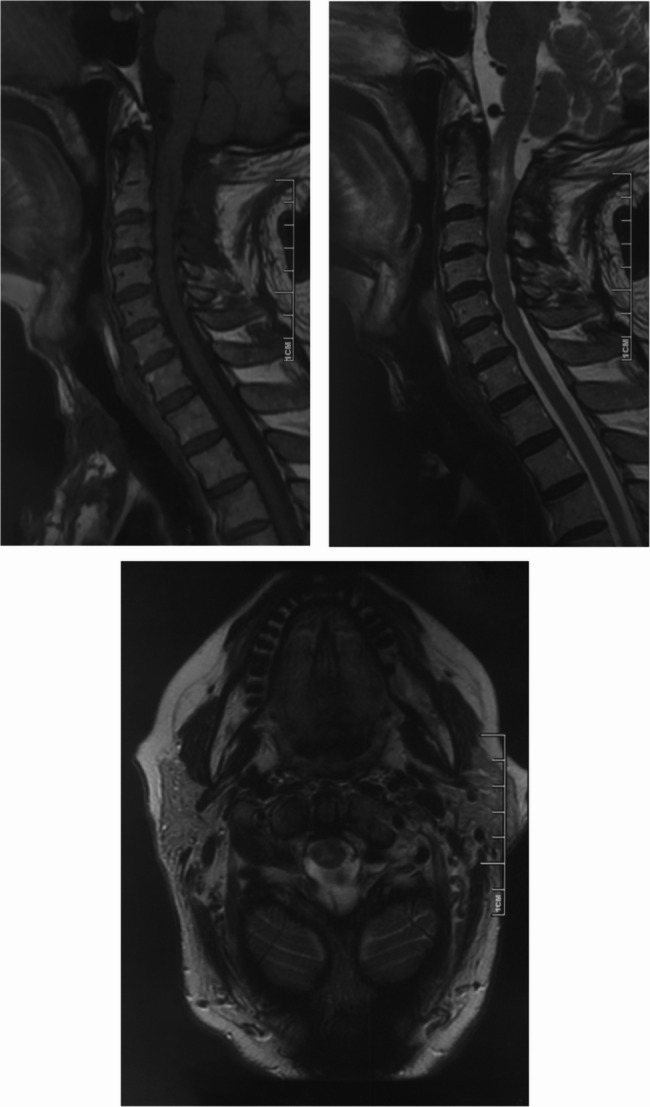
Postoperative MRI showing no evidence of tumor recurrence at the 7-month follow-up
